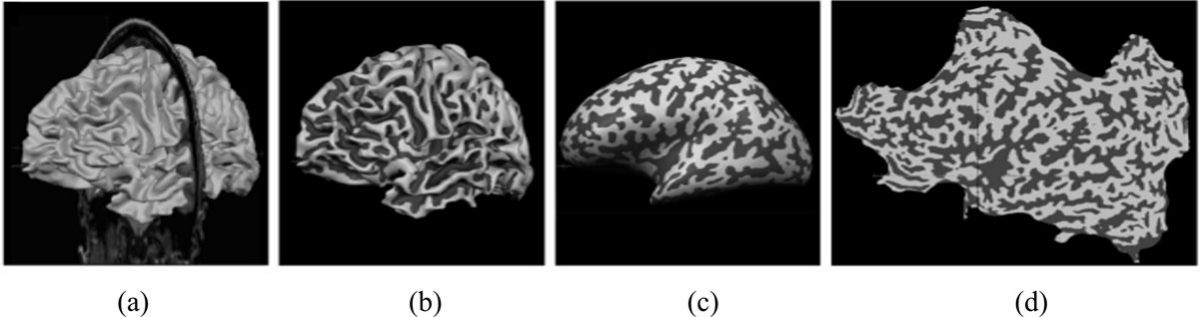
Flatmap generation from anatomical MRI. (a) reconstructed grey-white-matter interface (gwI) (b) overlayed curvature depicts gyri (light) and sulci (dark) (c) inflated gwI (d) flattened gwI.
